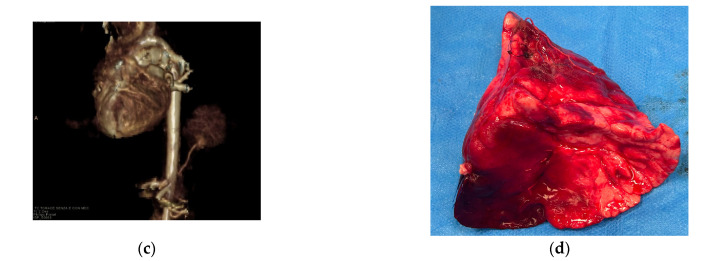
Intra-lobar bronchopulmonary sequestration. The Contrast-enhanced computed tomography (CECT) axial (a), coronal MPR reconstruction (b), and volume rendering reconstruction (c) images show a parenchymal consolidation located in the lower left lobe supplied by an afferent artery originating from the suprarenal tract of the abdominal aorta. Intraoperative findings confirmed the bronchopulmonary sequestration (d).
